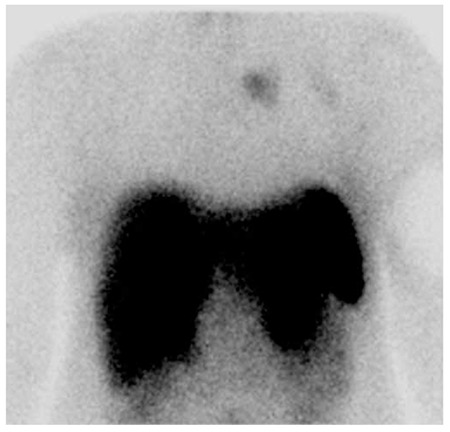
Technetium-99m-labeled octreotide acetate scintigraphy in the planar view of the thorax (anterior). This was performed 15 minutes after injection of 740 MBq technetium-99m-labeled octreotide acetate. There was an area of abnormal uptake in the left lung field
